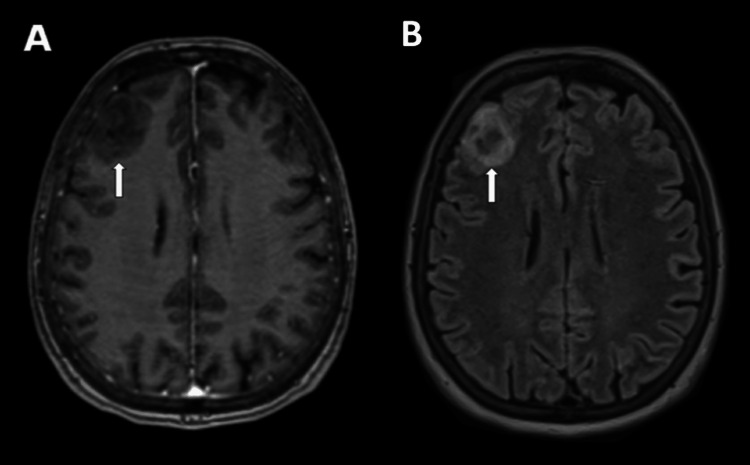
Axial MRI images on the day following admission showing no contrast enhancement (A), but perifocal edema in FLAIR (B) FLAIR: fluid-attenuated inversion recovery
